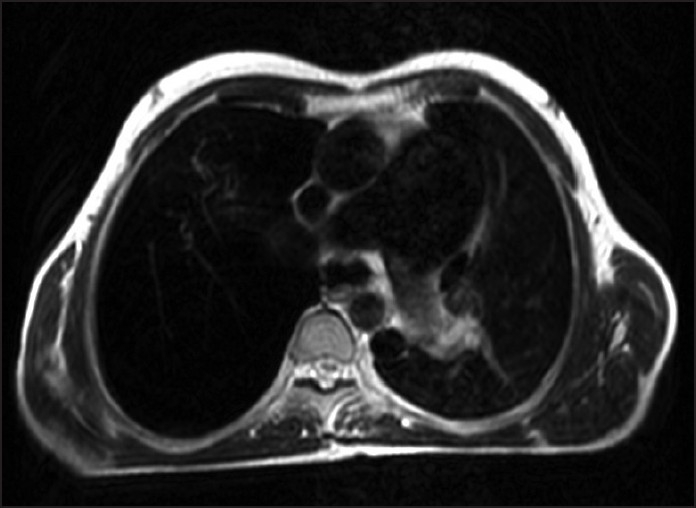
Axial T2-weighted MRI at carinal level shows a grossly enlarged main pulmonary artery, accessory hemiazygous and persistent left superior vena cava.
